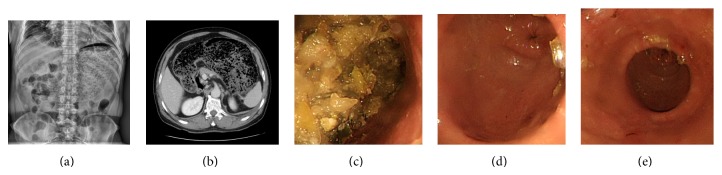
(a) Simple abdominal X-ray reveals a large amount of material in the stomach, suggesting severe gastric hypomotility. (b) Abdominopelvic computed tomography reveals a large amount of food material in the stomach, suggesting partial stricture in the postbulbar portion of the duodenum. (c) Endoscopic findings reveal large amounts of food stored in the gastric body and antrum. (d) The food was removed using a cap-fitted endoscope and a net over a period of days. (e) Peristaltic motion of the gastric antrum was normal and the pylorus opened and closed normally.
